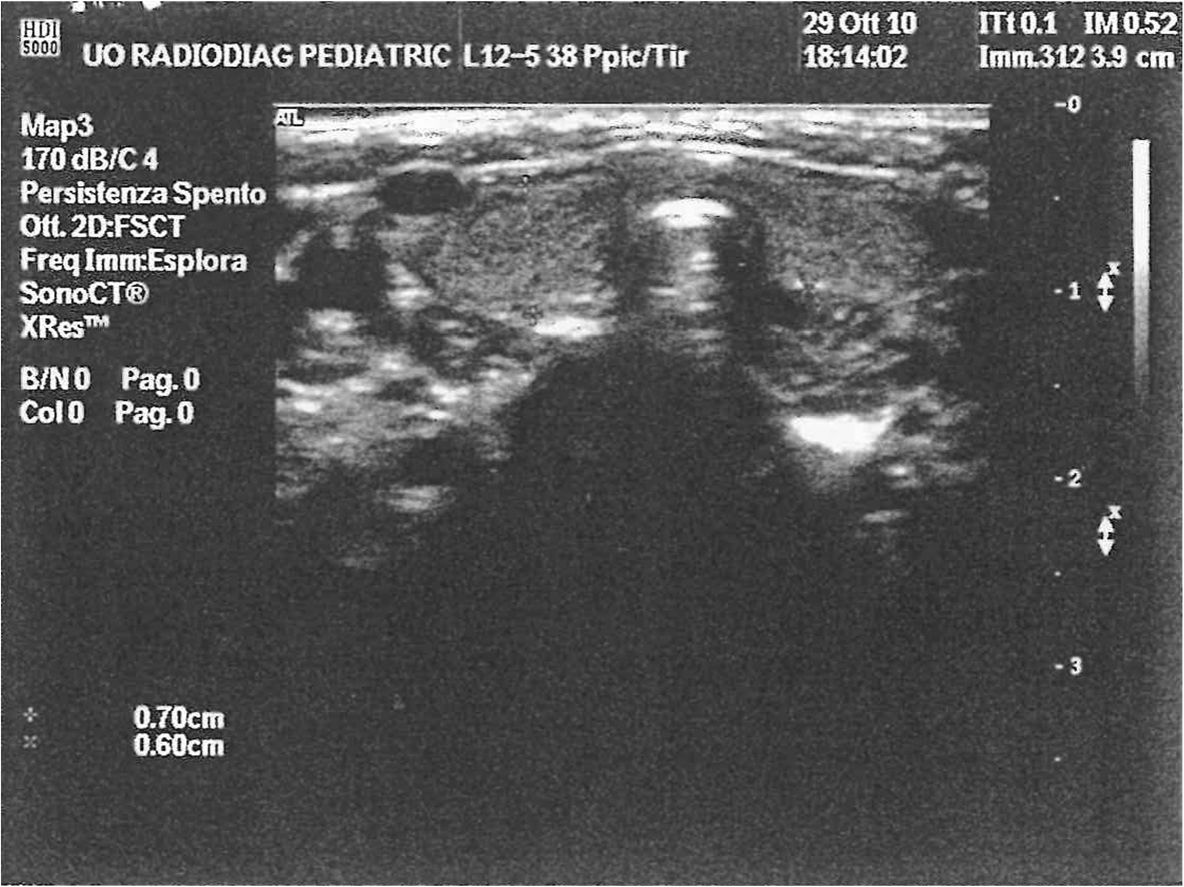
Ultrasound image of thyroiditis in a 6-month-old child.
